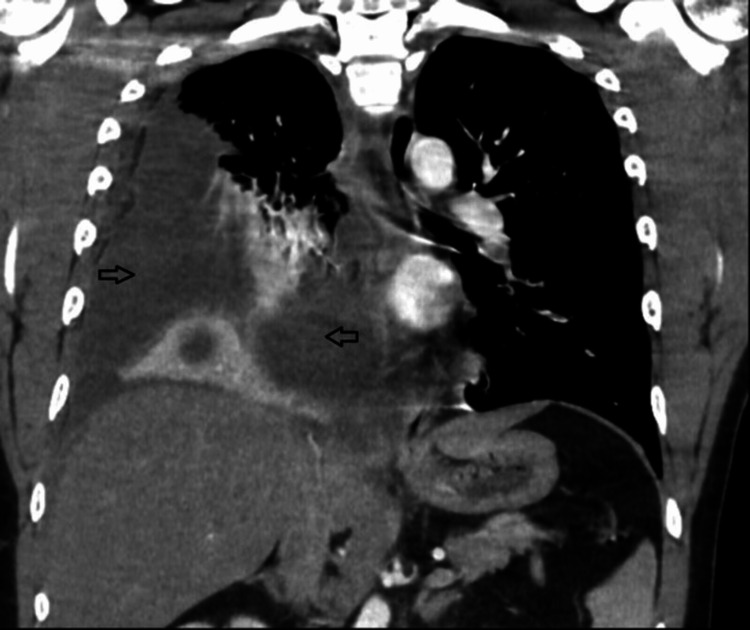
CT scan of the chest coronal view showing loculated pleural effusion (arrows) on admission
